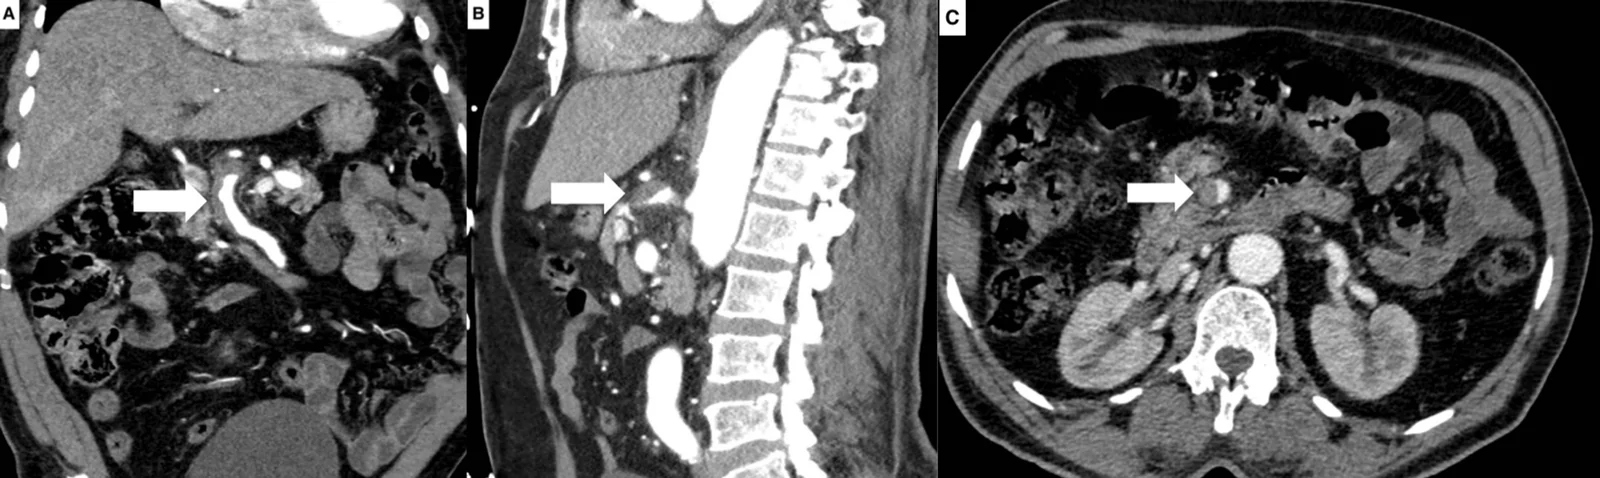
Follow-up CTA of the abdomen and pelvis obtained two days after the initial study, demonstrating a stabilized superior mesenteric artery dissection measuring approximately 4 cm in length. (A) Coronal, (B) sagittal, and (C) axial views are shown CTA: computed tomography angiography
